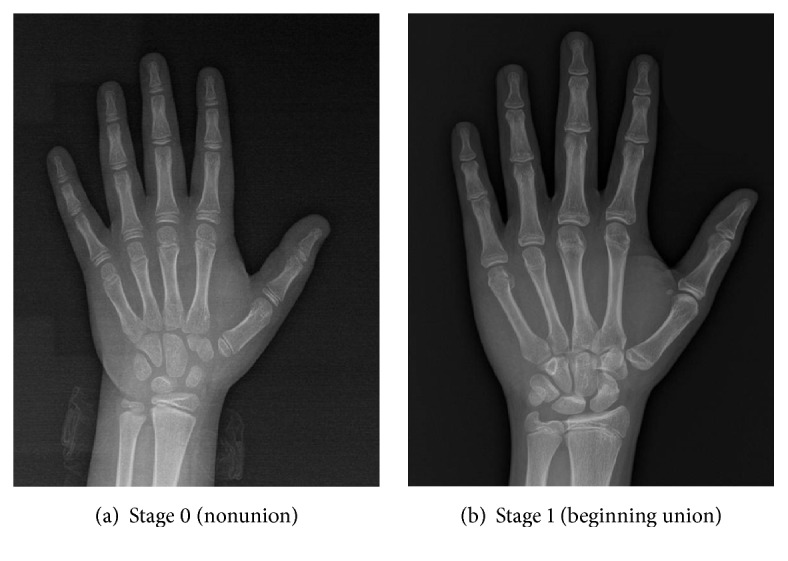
Radiographic images of hand joints showing stage 0 labeled as (a) and stage 1 labeled as (b).
